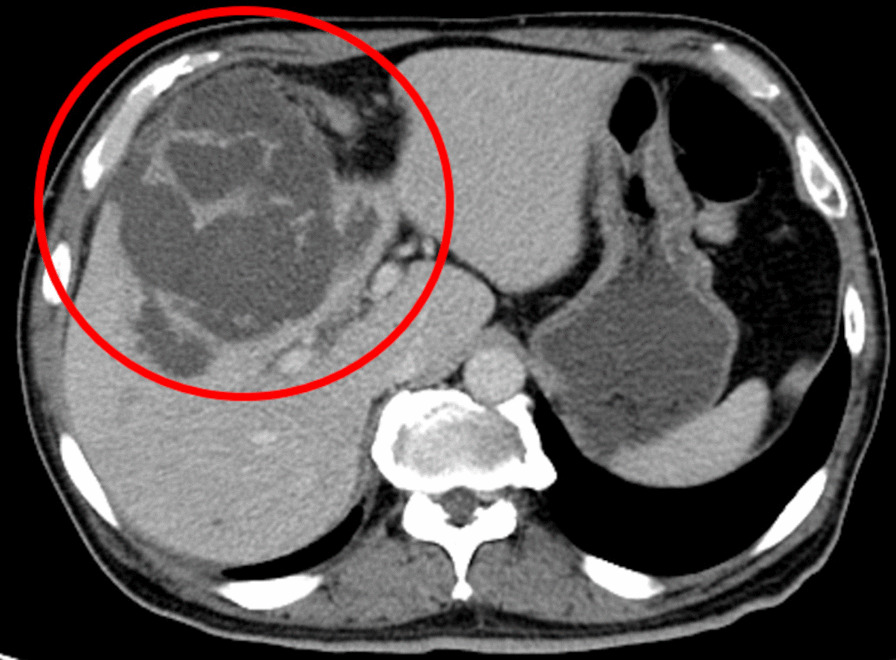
Contrast-enhanced abdominal CT demonstrated enlarged and multifocal gallbladder and abscesses around the gallbladder, some perforating into the right lobe of the liver and forming liver abscesses
